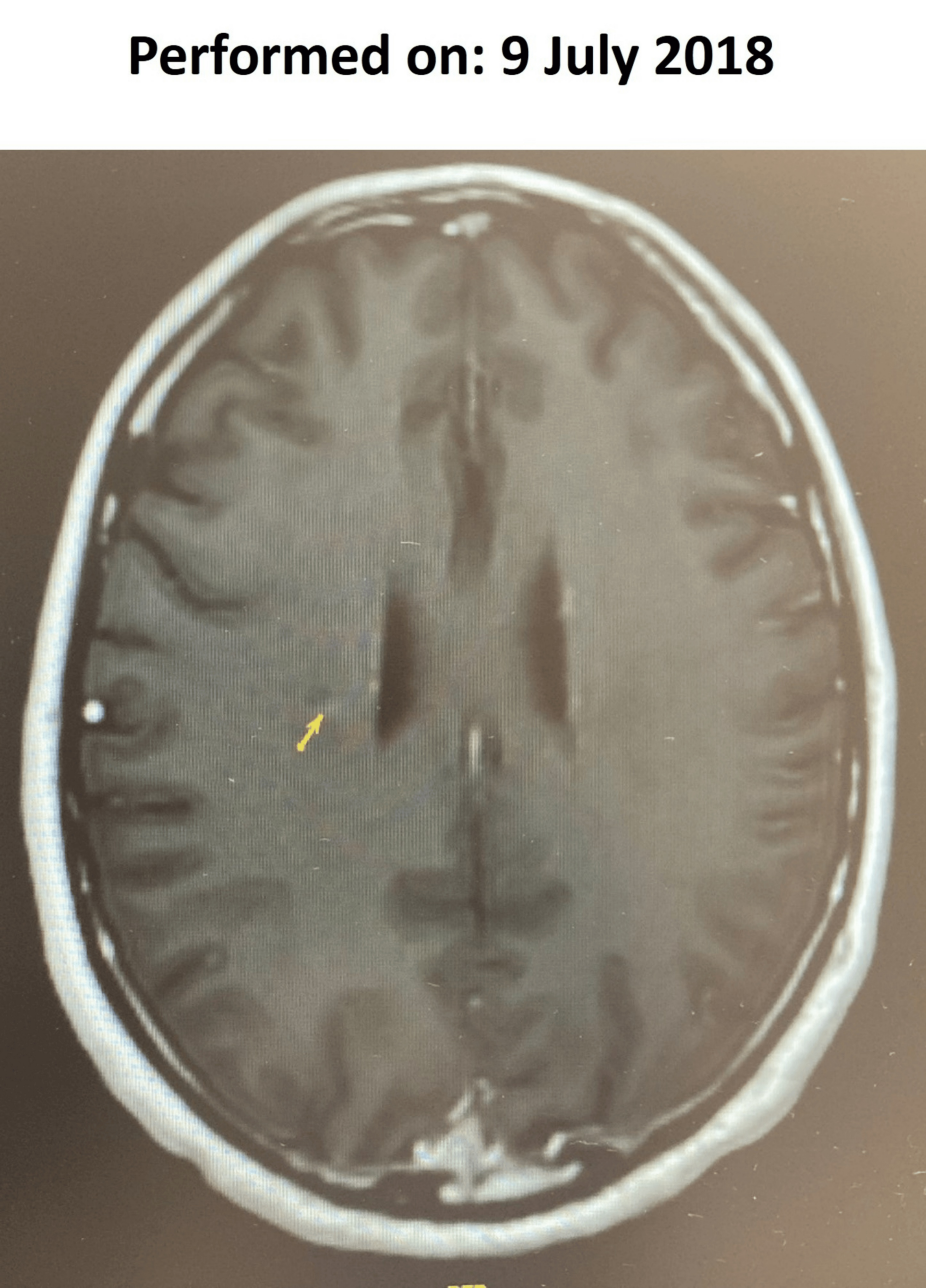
Subsequent cranial magnetic resonance imaging scan with contrast for Case 2
